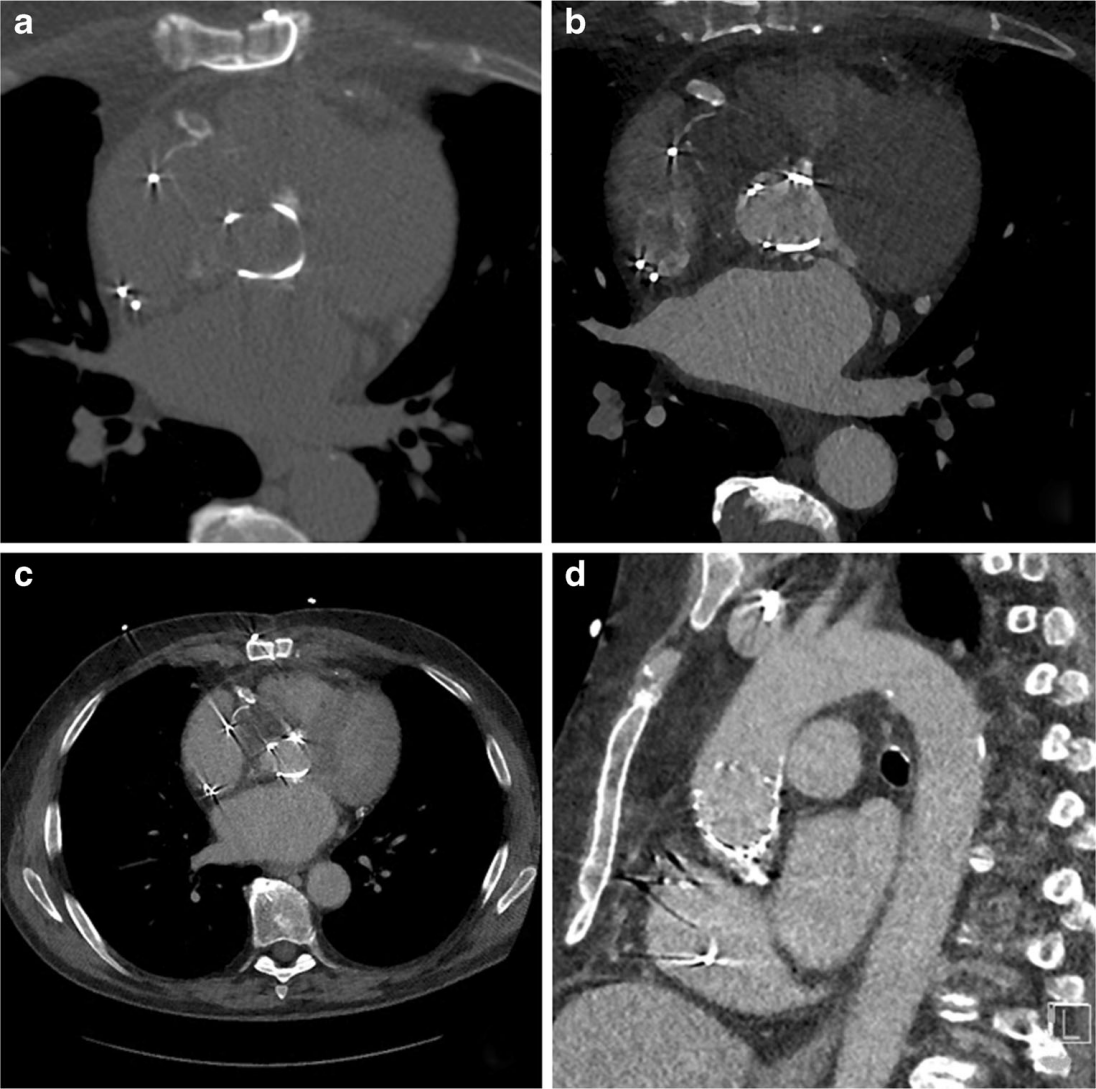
Image acquisition includes three sequential acquisitions: A non-contrast-enhanced scan (A); a contrast-enhanced arterial CT angiography (CTA) with reconstructions at each 5 % of the R-R interval (B); and a delayed high pitch CTA of the entire chest (C), which allows for evaluation of the complete thoracic aorta as shown on this sagittal view (D)
